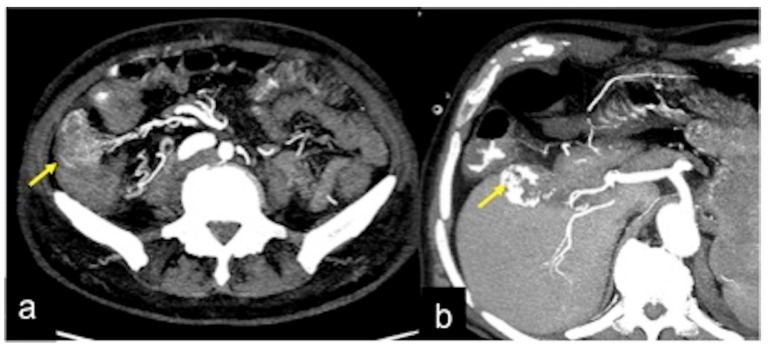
CTA multiphasic study of hepatic colon flexure angiodysplasia. In the arterial phase (a) and MIP reconstruction (b), it is possible to detect a haemorrhagic focus contiguous to a series of tortuous blood vessels in the colon wall (a,b, arrow).
